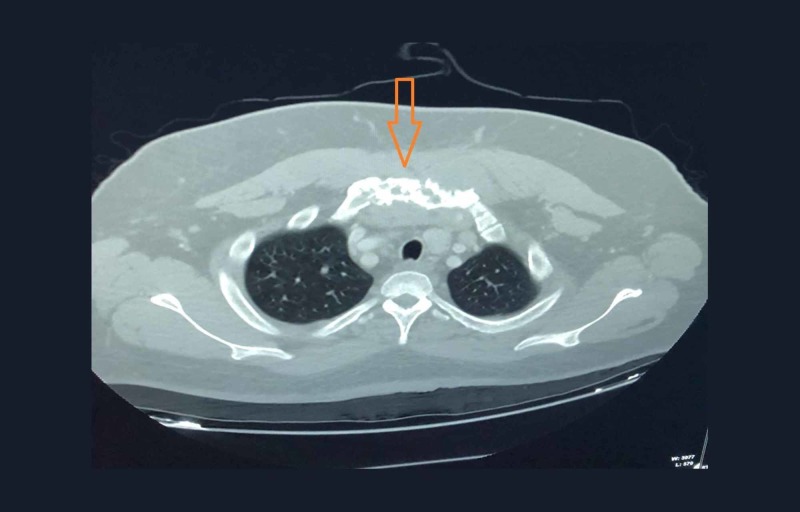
CT scan of the neck demonstrates an 8.6-cm anterior neck subcutaneous soft tissue mass extending into the anterior mediastinum through the sternum, with erosive changes visible in the sternum. The lesion is adjacent to the right common carotid artery and innominate vein, and surrounds the medial aspect of the clavicles bilaterally.
